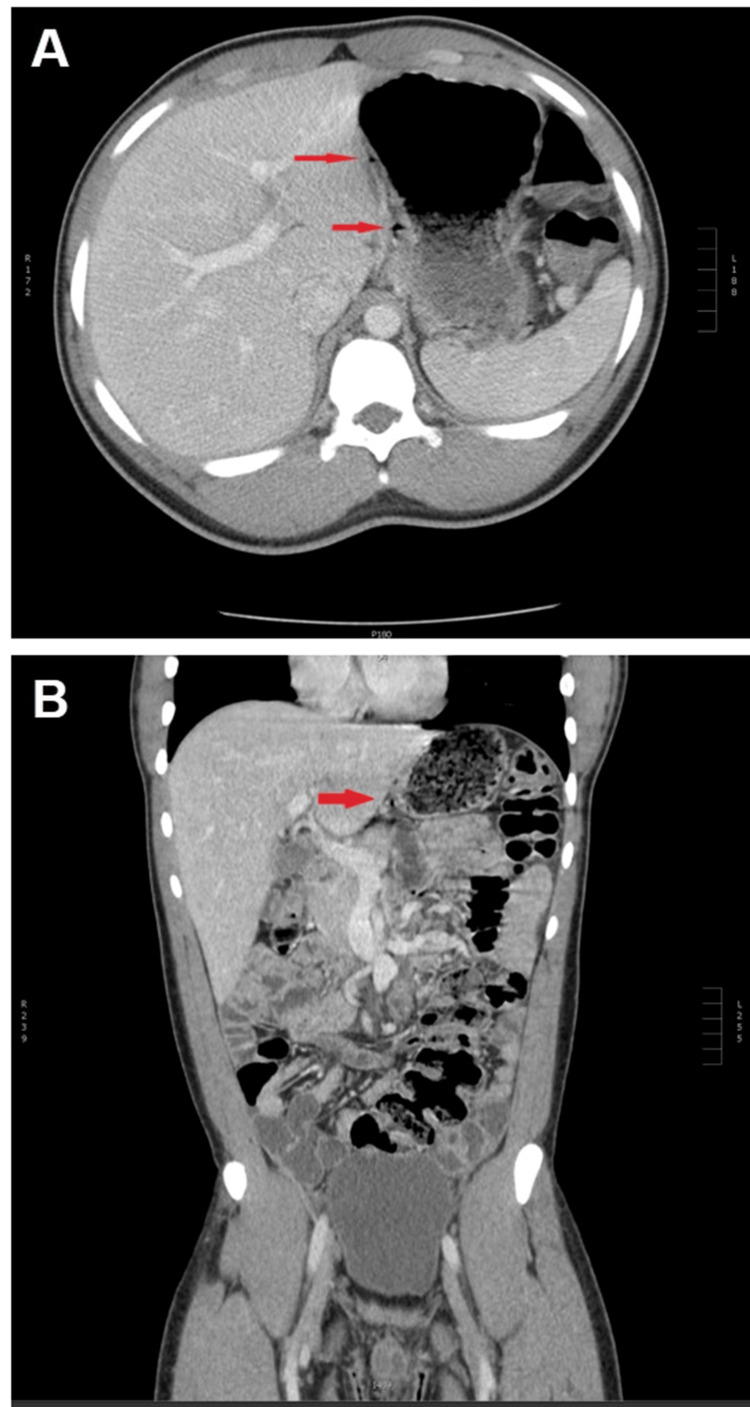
Images of the admission computed tomography scan with IV contrast of the abdomen/pelvis. (A) Axial and (B) coronal scans with arrows indicating tiny air density foci in the subcapsular region of the left lobe of the liver from the gastrohepatic ligament going medial to the wall of the stomach. This is a suboptimal view, as the CT scan was done in the portal venous phase instead of the preferred arterial phase. We believe this shows evidence of inflammation of the liver capsule despite not showing the characteristic liver capsule enhancement expected in an arterial phase scan.
